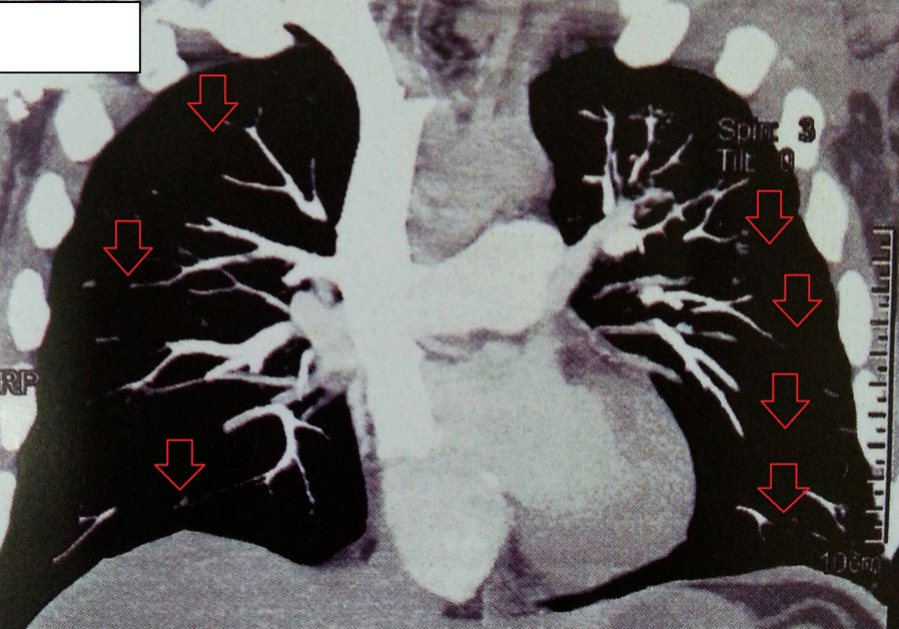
CT angiography (CTA) in the reported case with COVID-19. The figure shows acute pulmonary thromboembolism (PTE), supported by filling defects in lateral segment branch of right lower lobe (RLL) and posteromedial segments of left lower lobe (LLL). Arrows show the filling defects due to PTE
